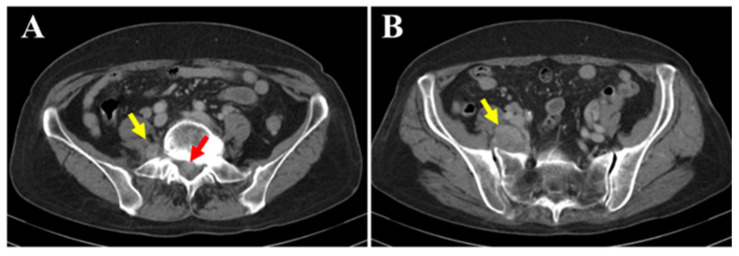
The patient was a 77-year-old woman who began experiencing pain from the right buttock to the lower limb three months prior to her first visit to our hospital. The patient initially consulted a local clinic, where imaging studies suggested a cauda equina tumor. A CT-guided biopsy was performed; however, the diagnosis remained challenging, and the patient was referred to our hospital. At her initial visit, she reported spontaneous pain and numbness extending from the right buttock to the posterior thigh and lower leg. There was no sensory dullness, the lower limb muscle strength was MMT grade 5, and no bladder or bowel dysfunction was observed. No café-au-lait spots were observed on the skin surface. Her medical history included hyperlipidemia and osteoporosis; however, she had no history of radiation therapy. Blood tests showed mildly decreased albumin (Alb 3.8 g/dL; normal range 4.1–5.1), slightly elevated creatinine (Cre 0.85 mg/dL; normal range 0.46–0.79), and mildly elevated IL-2R (597 U/mL; normal range 204–587), with all other values within normal limits. Contrast-enhanced CT (A,B) revealed a mass located both within the spinal canal (red arrow) and outside the intervertebral foramen (yellow arrow). No changes were observed in the surrounding bone.
